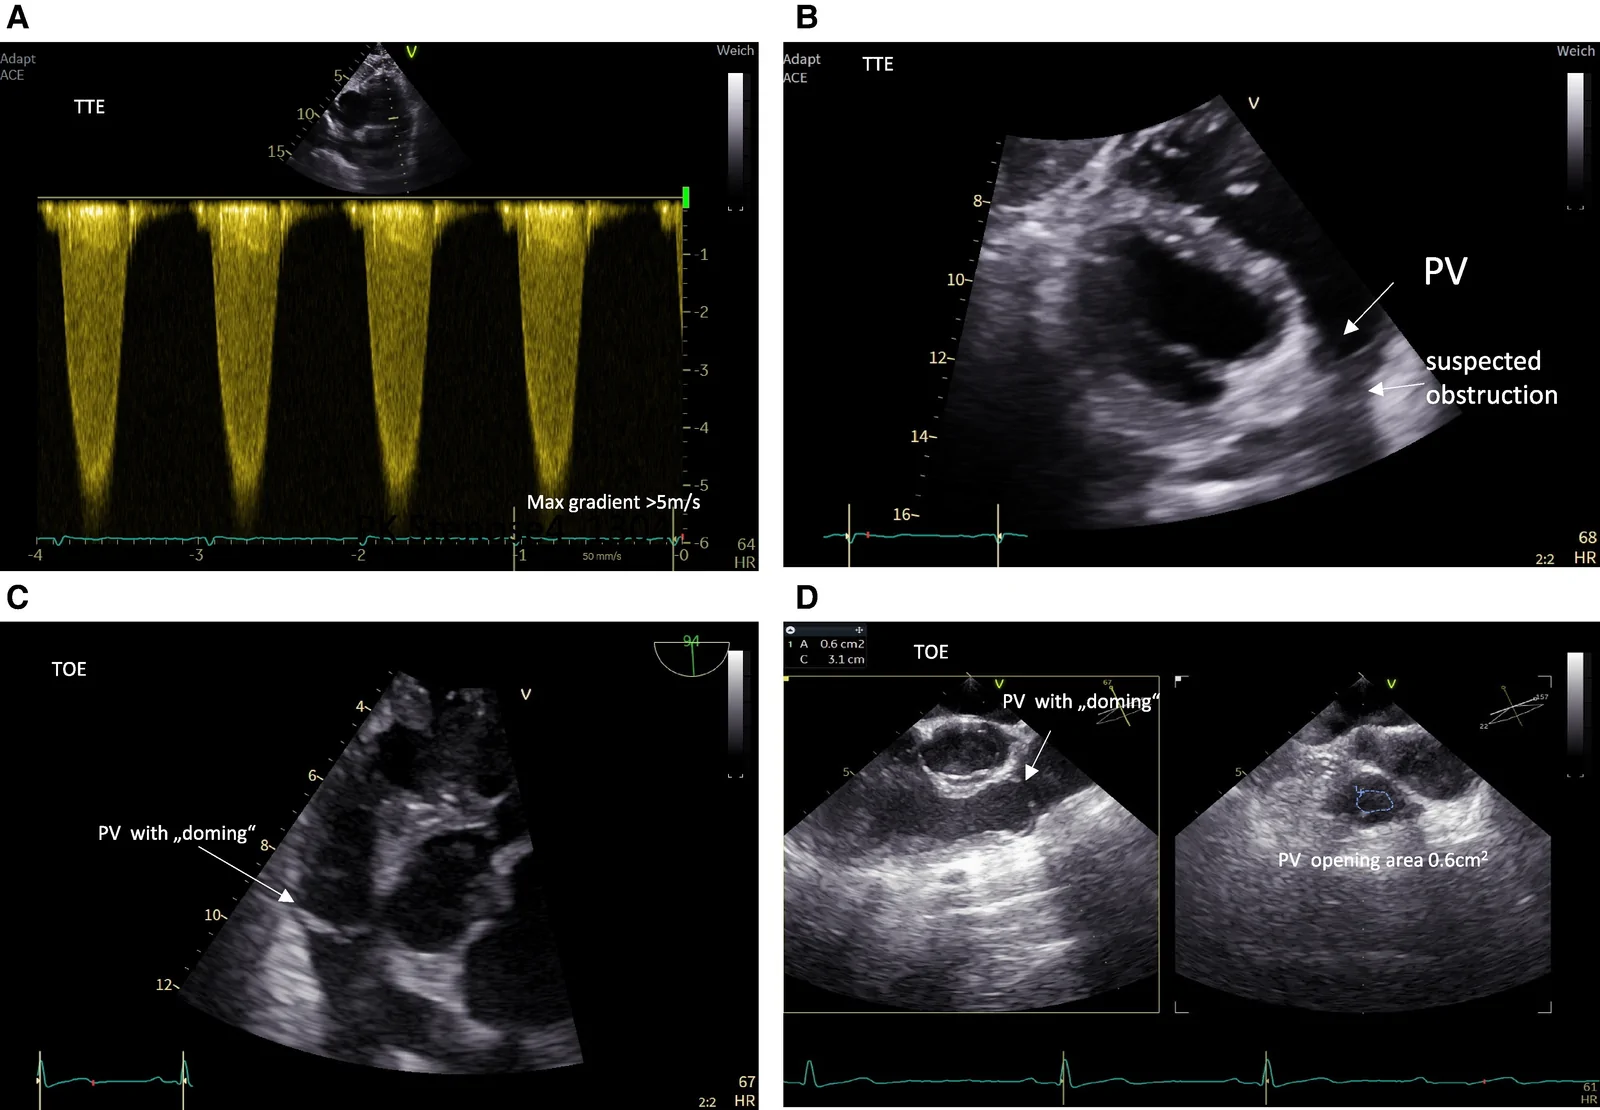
(*A*) Doppler gradient with maximum velocity of more than 5 m/s across the pulmonary valve was recorded. (*B*) Transthoracic echocardiographic image of pulmonary valve stenosis, unable to differentiate between supravalvular and valvular stenosis. (*C* and *D*) Initial transoesophageal echocardiography images clearly show valvular stenosis with doming, which mimics supravalvular stenosis, with a valve opening area of 0.6 cm^2^.
